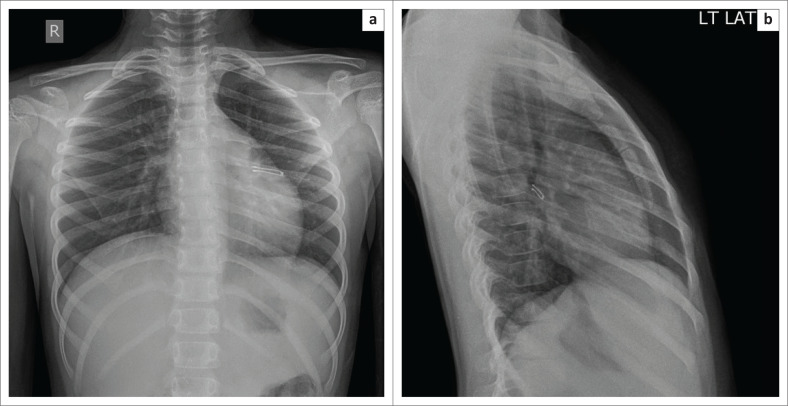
Frontal (a) and lateral (b) radiographs in a 3-year-old child who aspirated a light emitting diode bulb into the left main stem bronchus.
